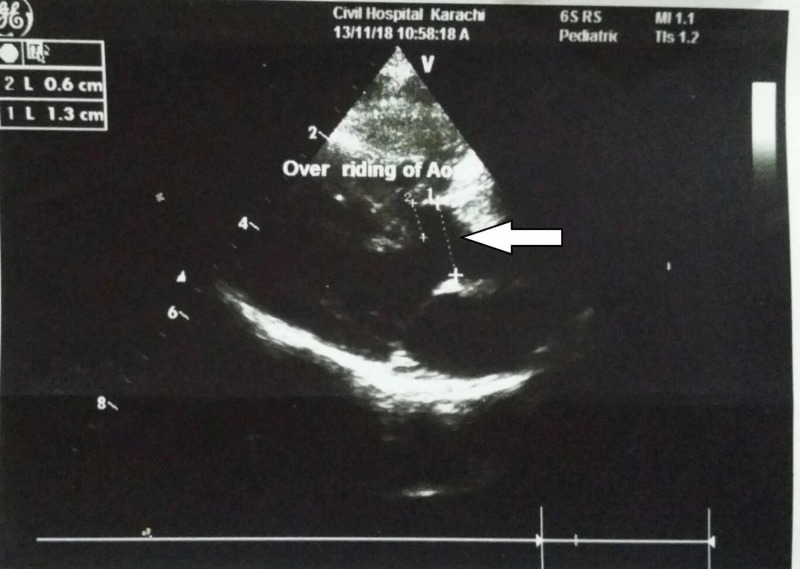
Transthoracic echocardiogram showing overriding of aorta
